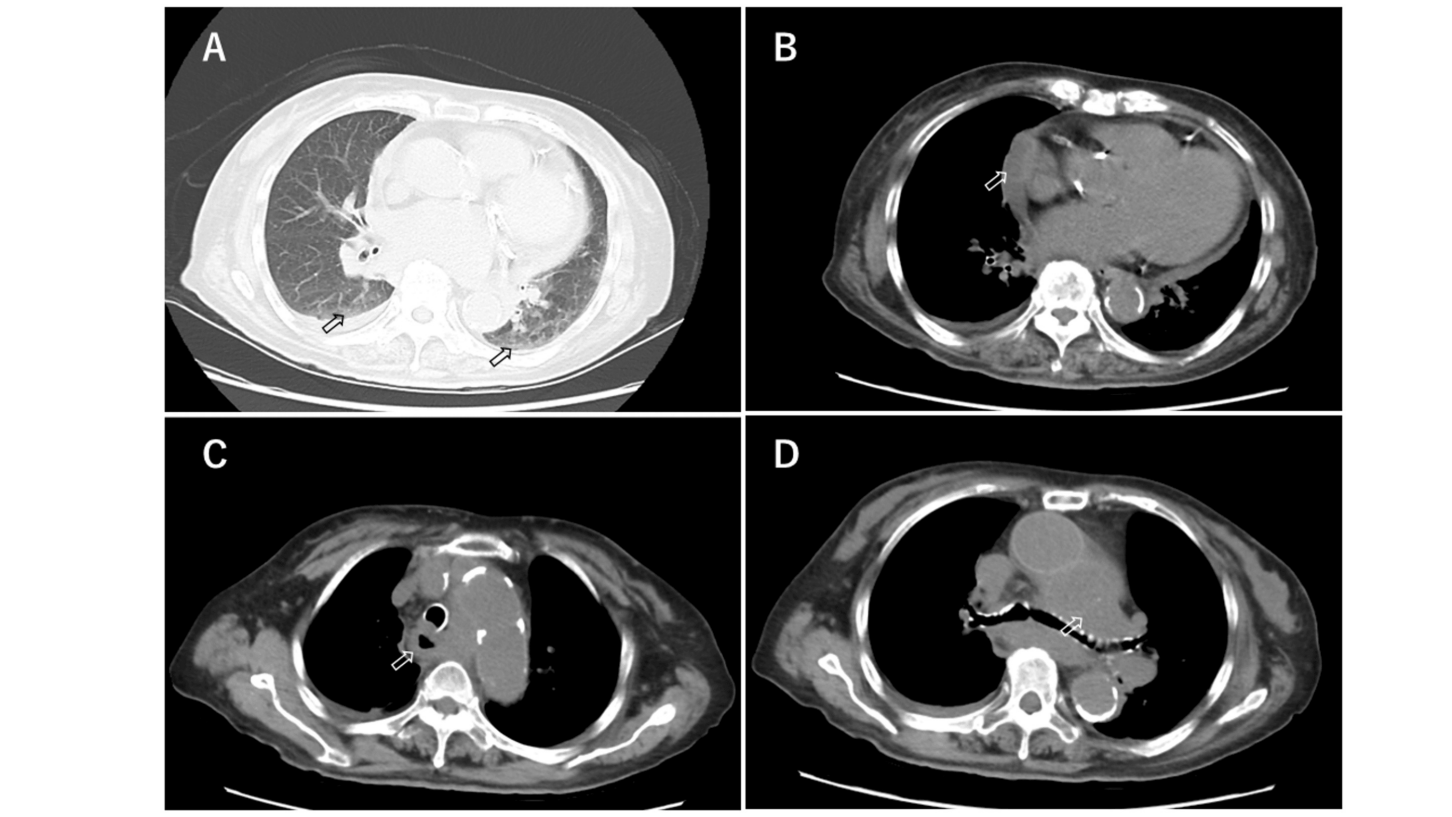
Chest plain computed tomography (CT) on the day of admission Chest plain CT on the day of admission when the patient manifested with fever, dyspnea, and chest oppression/pain showed ground glass opacity in the lingular segment of both lungs and dorsal inferior lobe (A, arrow), soft pericardial shadows (B, arrow), thickening of the esophageal wall (C, arrow), and mediastinal mass shadow (D, arrow). These findings raised a suspicion of esophageal cancer with its tracheal invasion and its lymph node metastasis in addition to pneumonia, initially. CT slice thickness: 5 mm.
